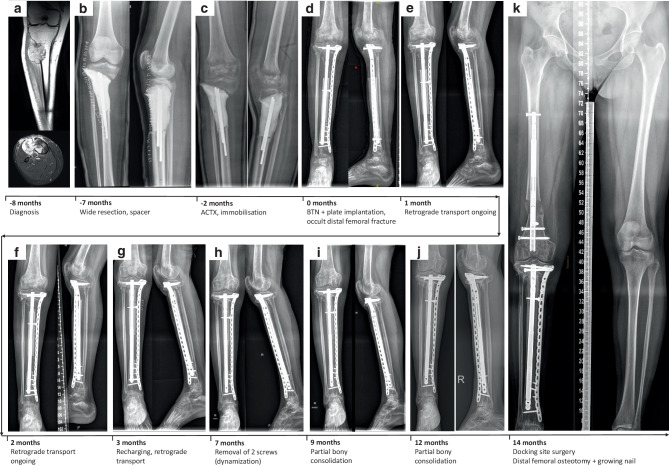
Clinical course of patient 3 (28-year-old female with malignant peripheral nerve sheath tumour (MPNST) of proximal right tibia. Magnetic resonance imaging (MRI) scan in coronal (top) and axial (bottom) plane showing the MPNST at the right proximal tibia (a). A.p. and lateral x-ray images following wide resection and spacer implantation (b) and during adjuvant chemotherapy with the spacer still in place (c). A.p. and lateral x-ray images taken directly following implantation of the bone transport nail (BTN) together with the plate (d), at 1 month (e), 2 months (f) and 3 months (g) following BTN implantation, with bone transport ongoing, at 7 months when two screws had just been removed for dynamization (h), at 9 months (i) and 12 months (j) with partial bony consolidation visible, and at last follow-up (14 months) when docking-site surgery had been carried out together with a distal femoral osteotomy and growing nail implantation for varus/flexion deformity correction (k). (*ACTX* adjuvant chemotherapy,* BTN* bone transport nail)
